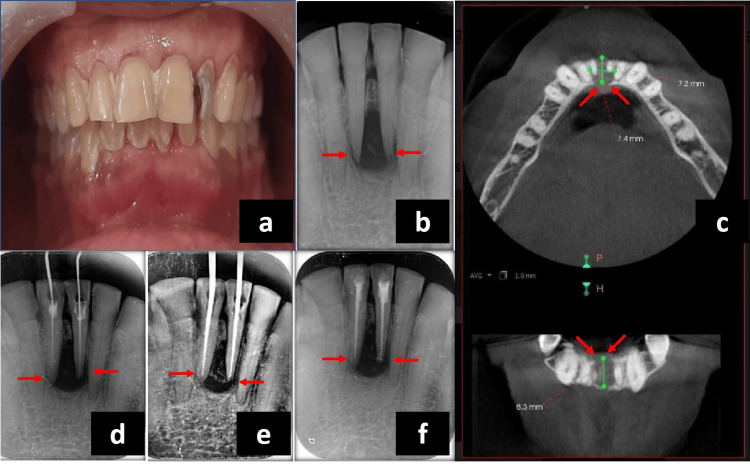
Pre-operative examination (a) Pre-operative intra-oral clinical picture. (b) Pre-operative intra-oral periapical radiograph showing large periapical radiolucency in the mandibular anterior region w.r.t. teeth 31 and 41. (c) Pre-operative cone-beam computed tomography images showing periapical radiolucency with loss of cortical plate on the lingual side in the axial section. (d) Intra-operative working length radiograph. (e) Intra-operative master cone radiograph. (f) Immediate post-obturation radiograph showing satisfactory obturation.
